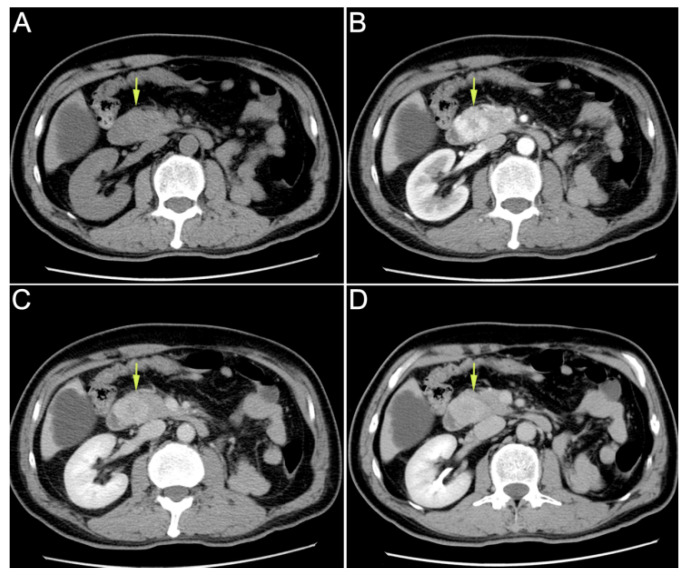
Enhanced upper abdominal computed tomography (CT) performed in the local hospital indicated ampullary mass. (A): plain scan phase; (B): arterial phase; (C): venous phase; (D): delayed phase. A 59-year-old male patient was hospitalized for “recurrent hematochezia for 8 days”. Enhanced upper abdominal computed tomography (CT), performed in the local hospital, indicated ampullary mass. On CT, the tumor showed uneven enhancement in the arterial phase, but the enhancement significantly weakened in the venous phase and delayed phase. Therefore, this imaging feature was significantly different from that in the primary ampullary tumor or pancreatic tumor. Duodenoscopy revealed duodenal papilla hemorrhage. Urinalysis showed no hematuria. After conservative treatment, the hemorrhage was not resolved. Then, the patient was transferred to our hospital for further treatment. He had received radical nephrectomy due to clear cell renal cell carcinoma (ccRCC) of the left kidney 16 years ago. The CT scan mentioned above revealed that his right kidney was normal. On admission, his laboratory tests results were as follows: leukocyte count of 3.9 × 10^9^/L; neutrophilic granulocytes percentage of 75%; C-reactive protein of 1.6 mg/L; hemoglobin of 102 g/L; total bilirubin concentration of 12.6 μmol/L; alanine aminotransferase of 16 U/L; aspartate aminotransferase of 19 U/L.
